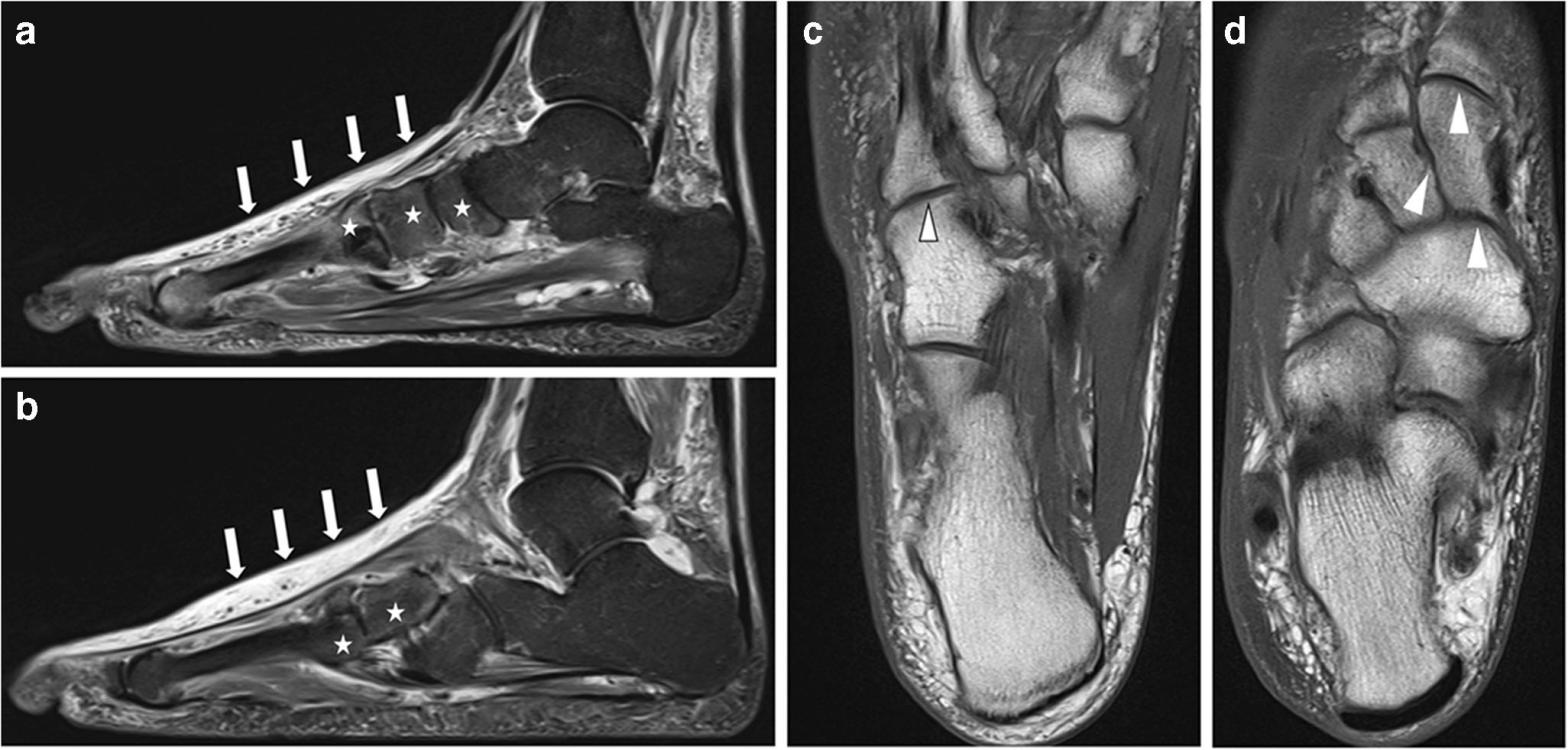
Eighty-four-year-old male patient (right foot) with Balgrist Score of 8 points and off-loading therapy of 64 days. a, b Sagittal STIR sequence showing severe soft tissue edema (white arrows) and some bone marrow edema (0–33%; asterisk) in region II and III. c, d No joint destruction is seen on transverse T1-weighted images (white arrowheads). No fracture was found
